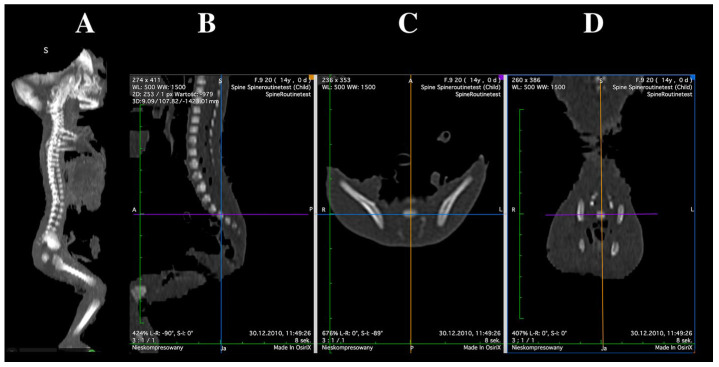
CT scan of a female fetus at 24 weeks of gestation: sagittal projection (A) and multiplanar reconstructions (MPRs) of the L5 vertebra in sagittal (B), transverse (C), and frontal (D) planes, obtained using OsiriX 3.9.
